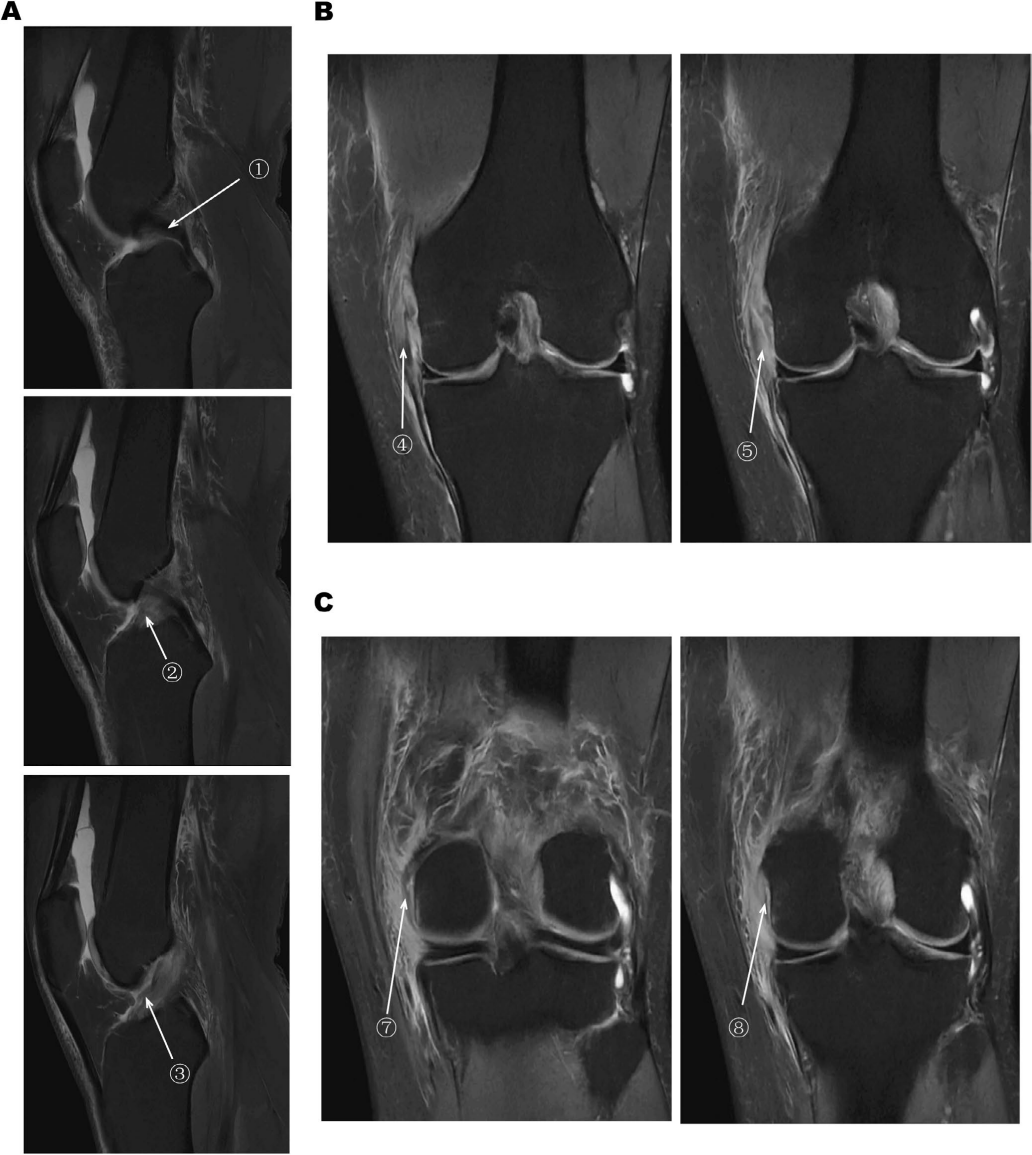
MRI images of knee ligament injury, Makers①–③: The posterior cruciate ligament is running normally with good tension, and the anterior crossover ligament (ACL) is torn. Markers④ and ⑤: Medial collateral ligament (MCL) femoral stop tear. Marker⑥ and ⑦: Posterior oblique ligament (POL) tear.
